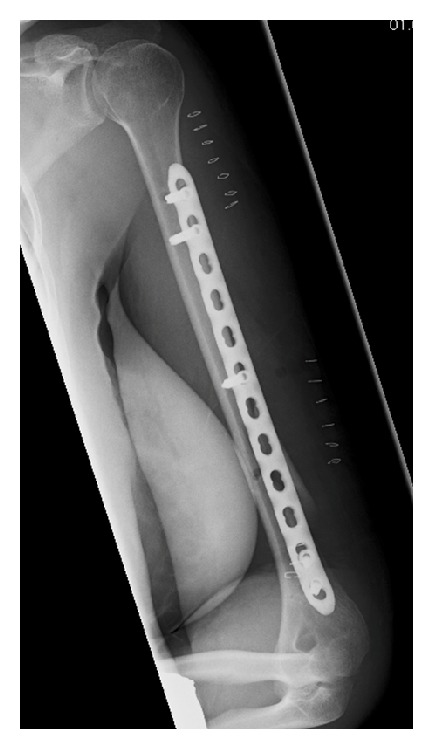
Postoperative radiograph shows the minimally invasive plate osteosynthesis and the skin staples.
